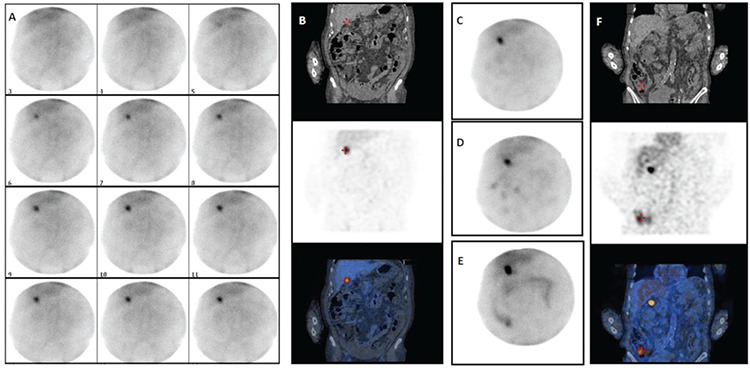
A 72-year-old male with intense activity in the upper right quadrant of the abdomen since the beginning of dynamic images (A). An early SPECT/CT imaging [(B) CT, SPECT, and fused SPECT/CT coronal planes] revealed that this area corresponded to the gallbladder. Therefore, imaging was continued to find the focus of the bleeding. While no additional finding was observed in the first-hour planar images (C), several areas of increased activity appeared in the third (D) and fifth hour (E) planar images. On a second late SPECT/CT, the bleeding was confirmed to originate from the ileocecal valve region [(F) CT, SPECT, and fused SPECT/CT coronal planes] SPECT/CT: Single photon emission computed tomography/computed tomography
